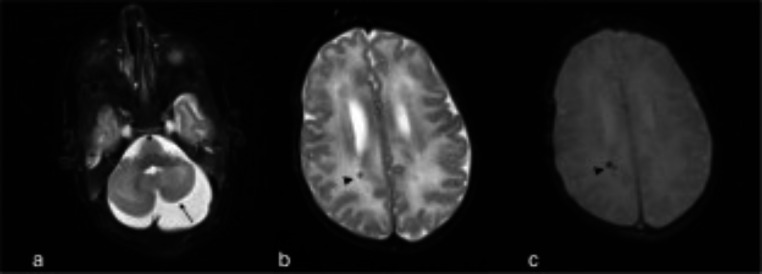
Clinical example of the use of brain MRI in CMV infection: Adapted from Vande Walle and colleagues [22]. Clinical example of the use of brain MRI in CMV infection: “Brain MRI in a 3-week-old male with confirmed cCMV infection, hematologic abnormalities, clinical signs of CMV infection, and hearing loss. Axial T2-weighted image (a) showing asymmetric, hypoplastic cerebellar hemispheres (arrow). Axial T2- (b) and T2*-weighted (c) images showing hypointense punctate lesions (arrowhead) near the posterior wall of the right lateral ventricle, with blooming artifact on the T2* image, representing calcifications.” Figure taken from Fig. 2 [22] in Vande Walle C et al., 2021, Eur Radiol. Oct;31 [10]:8001-801 [22]. Copyright 2021. European Society of Radiology. Reprinted with permission from Springer Nature (License : 5,752,021,193,227)
